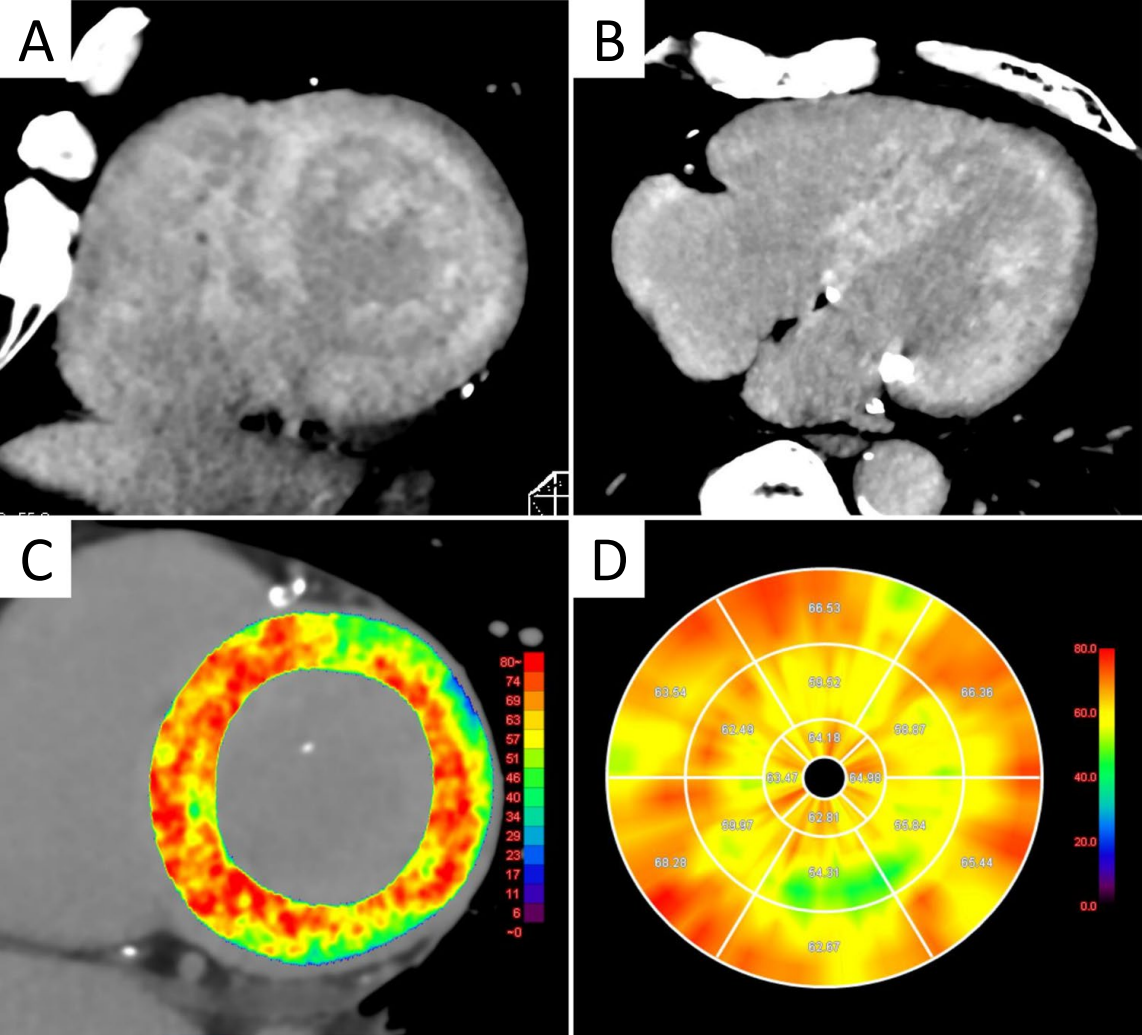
CT findings in ATTRwt-CM. A man in his 70 s with wild-type transthyretin cardiac amyloidosis (ATTRwt-CM). The patient was scheduled for transcatheter aortic valve replacement (TAVR) due to severe aortic stenosis. Preprocedural planning CT with additional late iodine enhancement (LIE) imaging revealed diffuse LIE in the left ventricular (LV) wall (A, B). CT-derived extracellular volume (ECV) maps demonstrated markedly elevated ECV values (yellow to red areas in C, D). Subsequent evaluation confirmed concomitant ATTRwt-CM
